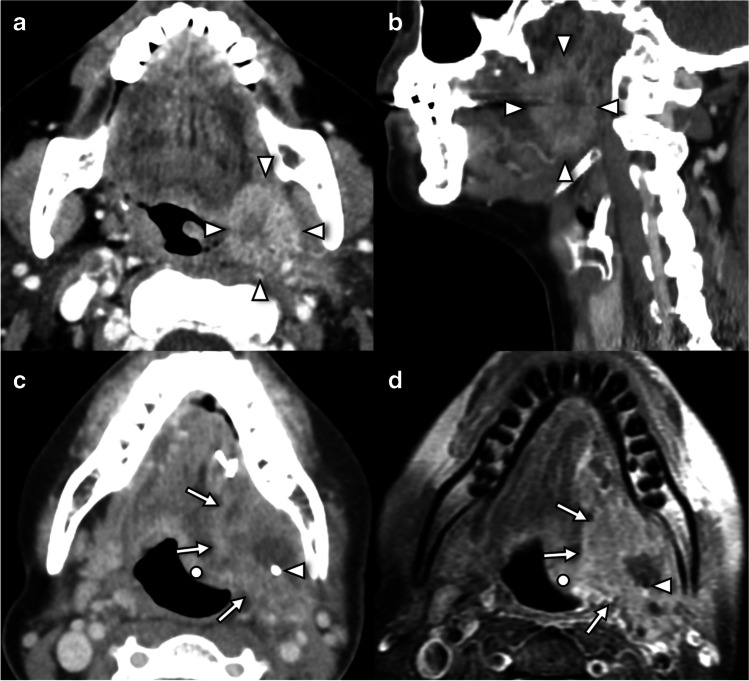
Lesions involving the sublingual segment of the hypoglossal nerve. a Axial contrast-enhanced CT in a 61-year-old female smoker with left neck pain and swallowing difficulty reveals a centrally necrotic, enhancing and infiltrative mass in the left glossotonsillar sulcus (arrowheads) consistent with squamous cell carcinoma. b Sagittal contrast-enhanced CT in the same patient as a demonstrates the mass (arrowheads) occupies the expected location of the hypoglossal nerve at the junction of carotid and sublingual space segments. c Axial contrast-enhanced CT in a 65-year-old male smoker status post partial glossectomy, neck dissection, and radiotherapy for left oral tongue SCC demonstrates a bulky, necrotic recurrent mass (arrows) around surgical clips (arrowhead) and accompanying left tongue ptosis (dot). d Axial contrast-enhanced T1W in the same patient as c better demonstrates extent of soft tissue involvement by recurrent tumor (arrows) around the surgical resection clips (arrowhead) with associated denervation ptosis (dot)
